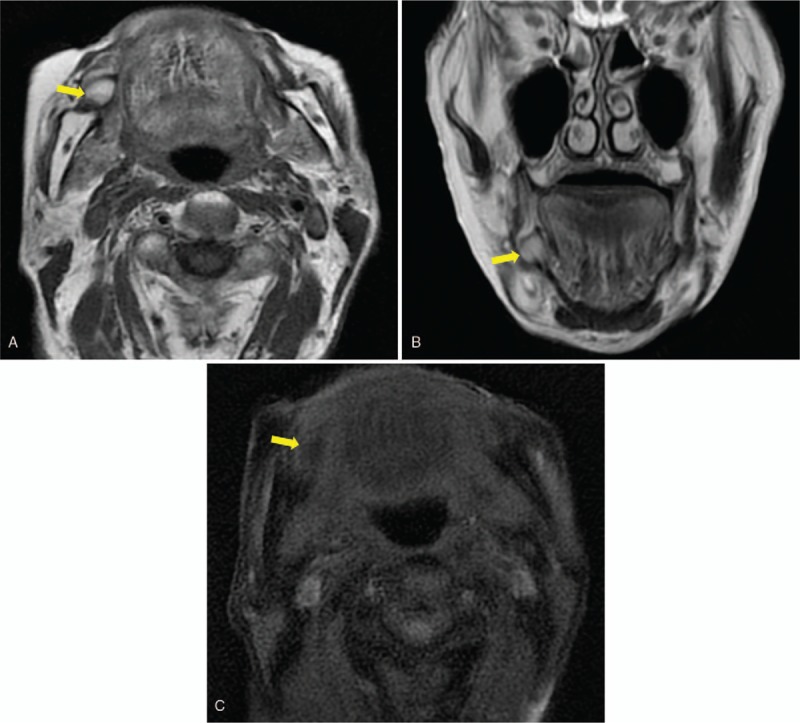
Axial and coronal MRI images showing a well-circumscribed lesion at the right buccal mucosa. The mass revealed high-signals in both T1-weighted (A, arrow) and T2-weighted images (B, arrow) and low-signals in fat-suppression T1-weighted images (C, arrow). MRI = magnetic resonance imaging.
